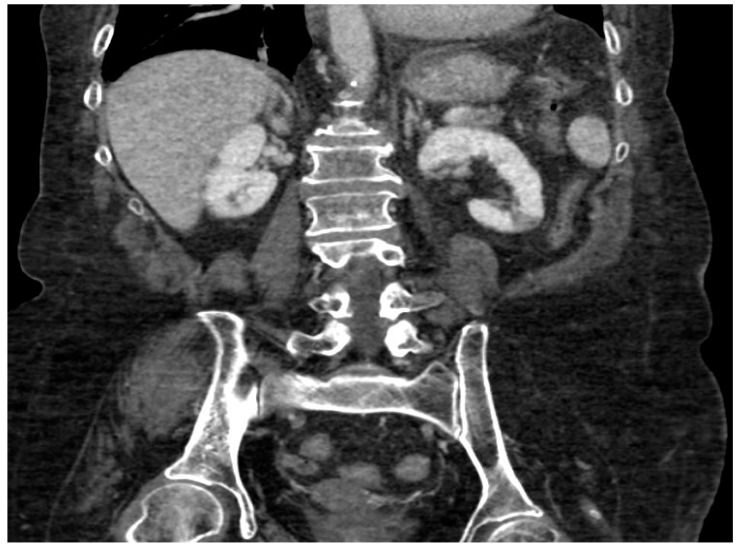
CT angiography, coronal planes, portal phase. Focal ischemia of the left renal parenchyma may be noted in the lower polar location.
